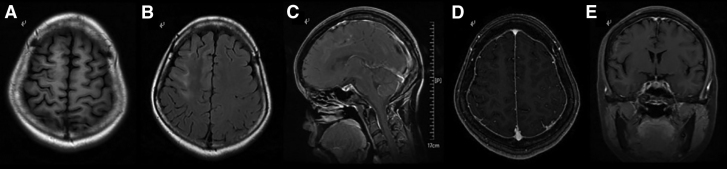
(A) The T1WI sequence of the cranial transverse section; (B) The T2WI sequence of the transverse craniocerebral section; (C) The T1WI sequence enhancement of the sagittal position; (D) Transverse enhancement of the T1WI sequence in cranial magnetic resonance imaging; (E) Coronal enhancement of the T1WI sequence in cranial magnetic resonance imaging. T1WI = T1-weighted imaging, T2WI = T2-weighted imaging.
